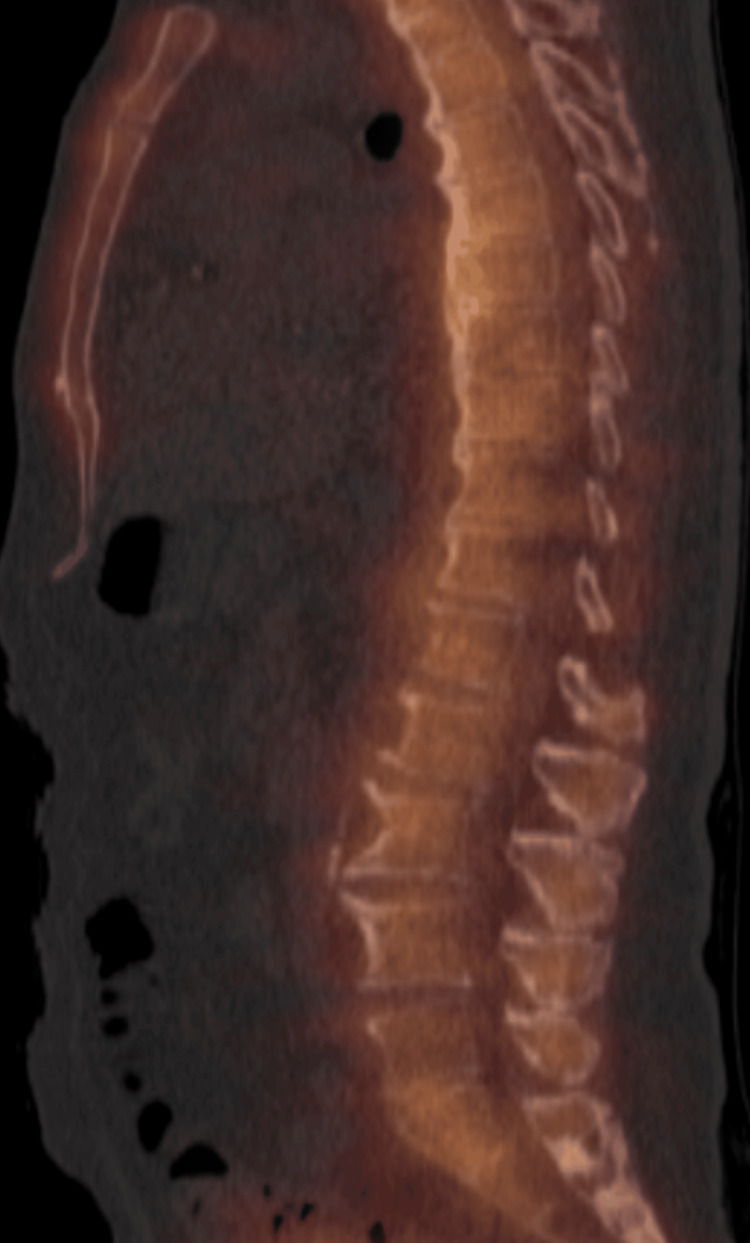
SPECT CT sagittal cut showing diffusely increase uptake along the anterior thoracic spine, reflecting DISH SPECT CT: single-photon emission computed tomography and computed tomography, DISH: diffuse idiopathic skeletal hyperostosis
